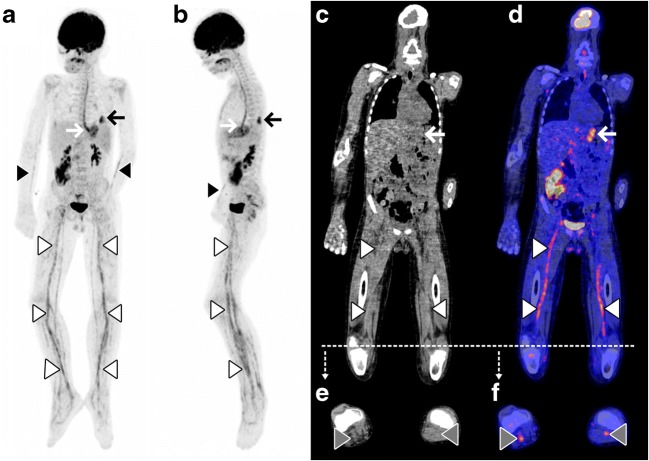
A 9-year-old boy was admitted to the hospital with general malaise present for 10 days, high fever (39.5 °C), painful legs, and bloody diarrhea. Physical examination showed increased respiratory effort, hepatomegaly, ascites, and erythema on the hands and feet. Laboratory testing showed a CRP of 307 mg/L, leukocyte count of 24.3 × 109/L, and erythrocyte sedimentation rate of 55 mm/h. MRI of the head and neck, chest radiography, and abdominal ultrasonography did not reveal any cause of fever, and microbiological cultures were negative for infection. As the patient did not respond to antibiotic treatment, corticosteroid treatment was also started. The symptoms improved, but the exact cause of the symptoms remained unknown, and FDG-PET/CT was performed. Coronal and sagittal maximum intensity projection FDG-PET (A and B), low-dose CT (C) and fused FDG-PET/CT (D), axial low-dose CT (E), and axial fused FDG-PET/CT (F) showed increased FDG uptake in the major arteries of the arms (black arrowheads) and legs (white arrowheads), including the popliteal artery in both knees (gray arrowheads). Based on these findings, the patient was diagnosed with polyarteritis nodosa. As collateral findings, increased FDG uptake in the esophagus due to irritation of a nasogastric feeding tube (white arrow) and a small infectious process in the lower lobe of the left lung (black arrow) were found.
